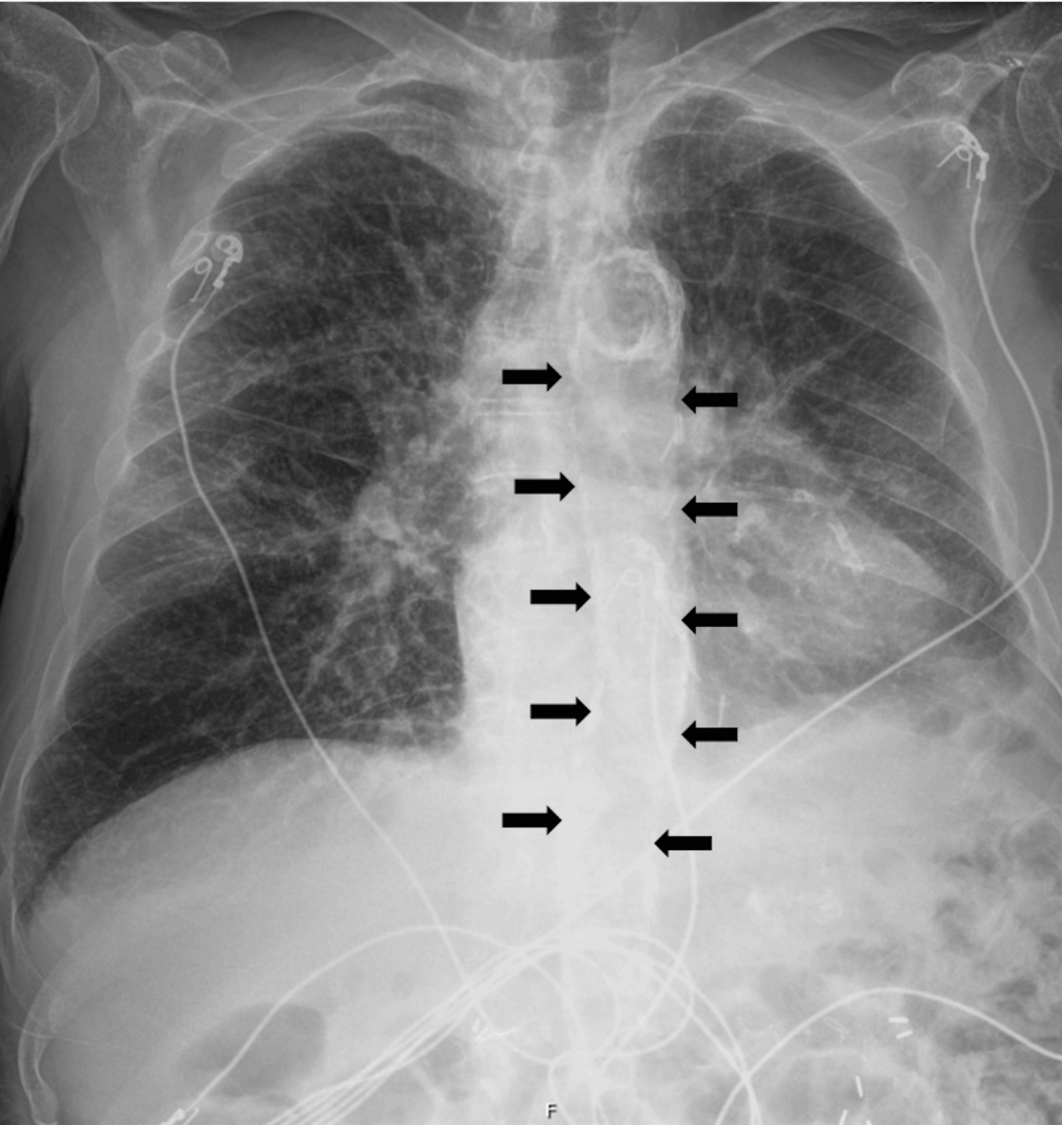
This image shows a posterior-anterior (PA) chest X-ray. There is extensive calcification of the aorta (black arrows).
